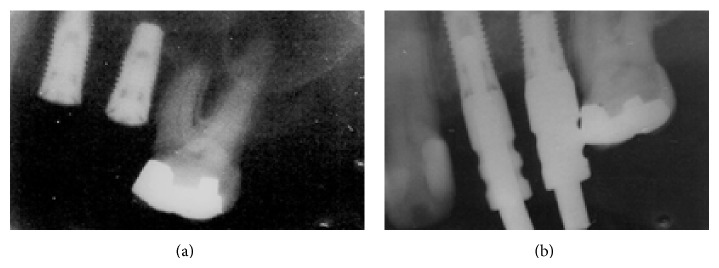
Periapical radiograph four months after surgery (a) and connection of the abutments (b) (March 2009).
